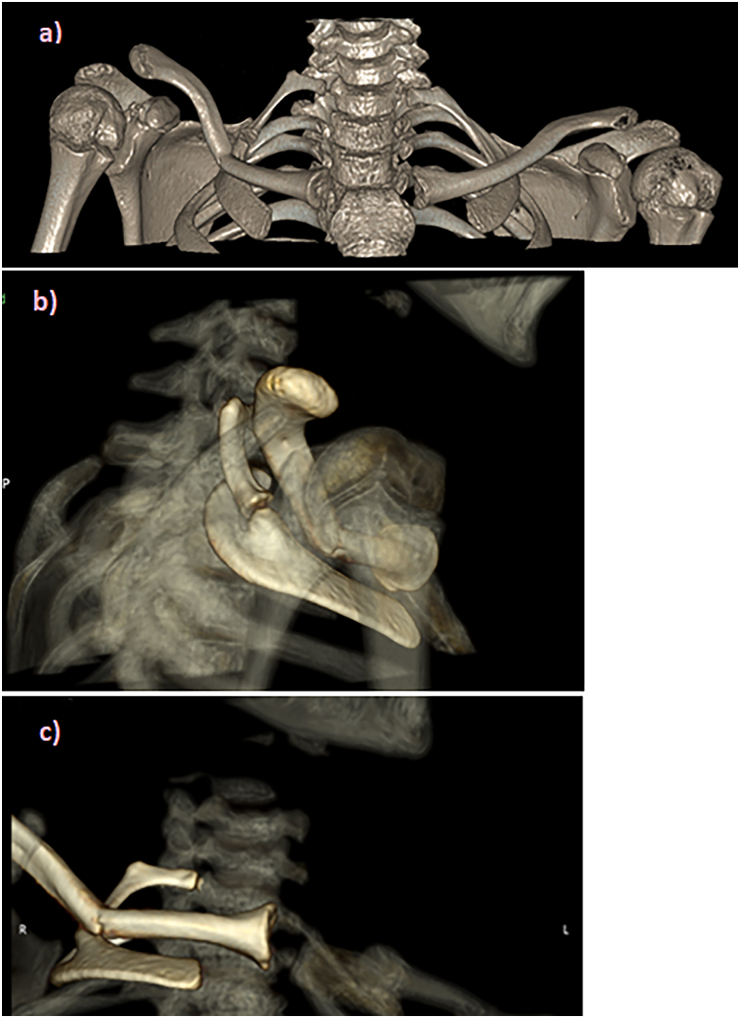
Three-dimensional computed tomography reconstruction of (a) both clavicles, (b) postero-lateral view the right clavicle and (c) anterior view of the right clavicle.
